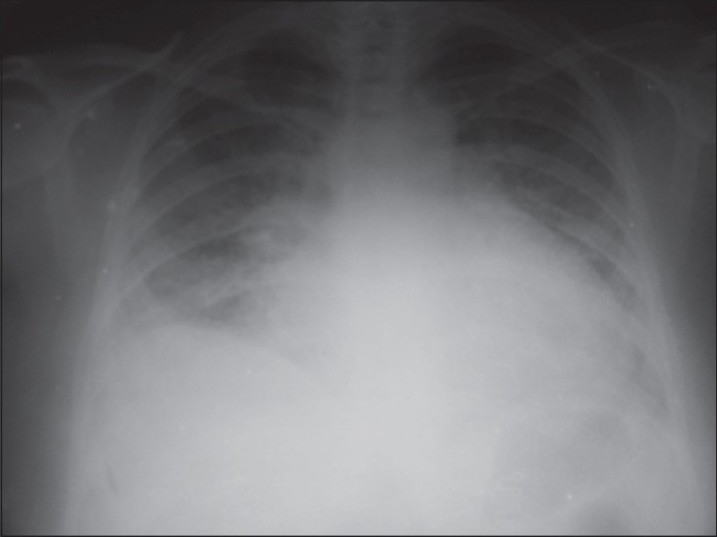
Chest X-ray at ICU admission
